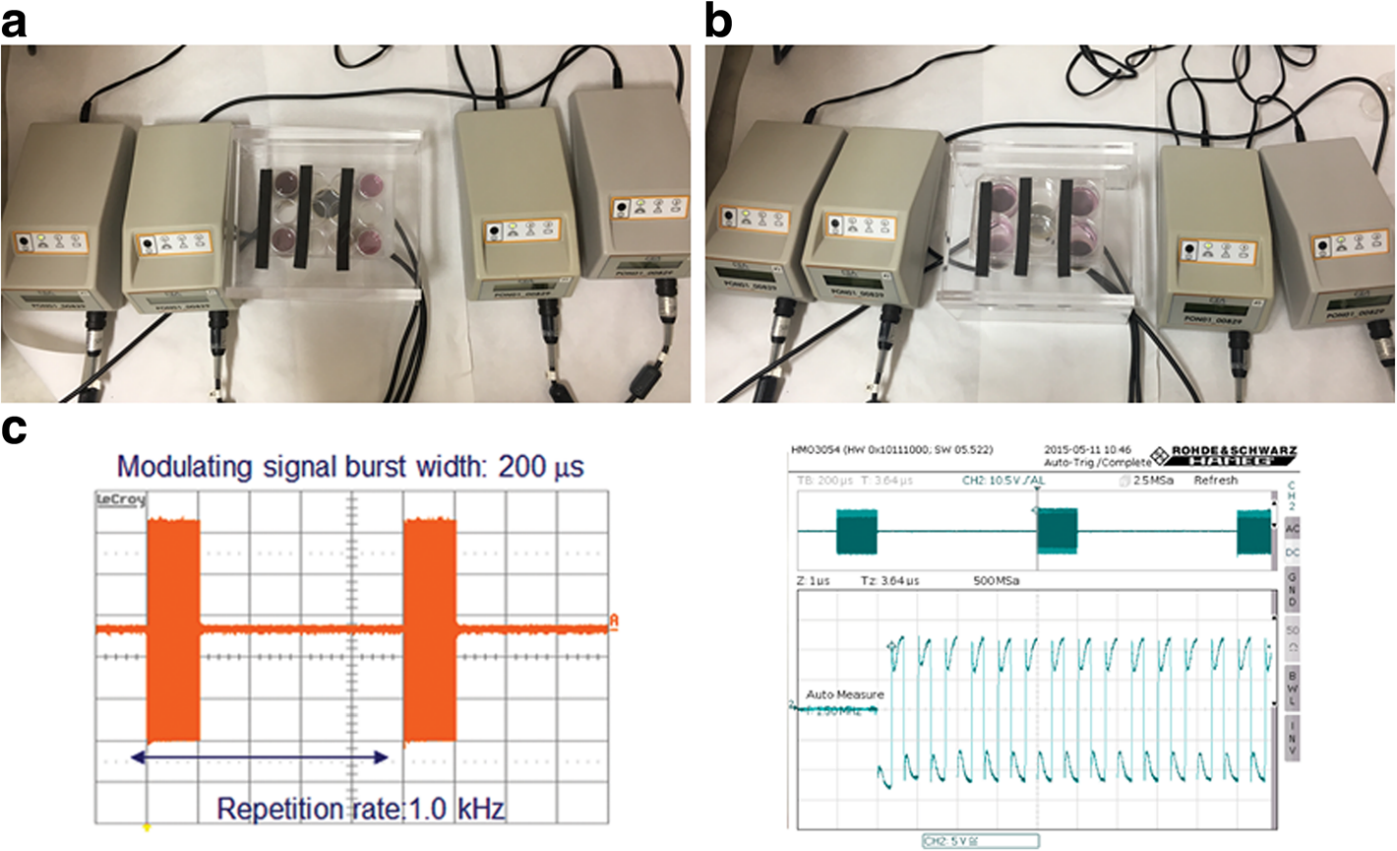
LIPUS set-up experiment. Upper panel: LIPUS transducer device. Bottom panel: characteristic of the ultrasound signal: 200 μs burst of 1.5 MHz sine waves repeated at 1 kHz (c). MDA-MB-231 cells were seeded in 12-well plates employing four wells/plate. Two wells were placed on swich-on transducer receiving LIPUS stimuli to obtain LCM the other two were placed on swich-off transducer and used as untreated group to collect CM. LIPUS treatment was performed for 20 min/day for 10 days (a). MDA-MB-231 cells were seeded in 6-well plates using four wells/plate. After 24 h, a transwell insert was inserted into each well and Raw264.7 cells were seeded in the upper compartment. Two wells were placed on swich-on transducer receiving LIPUS stimuli to obtain LIPUS_CC group, the other two were placed on swich-off transducer and used as untreated to obtain Untreated_CC group. LIPUS treatment was performed for 20 min/day for 10 days (b)
